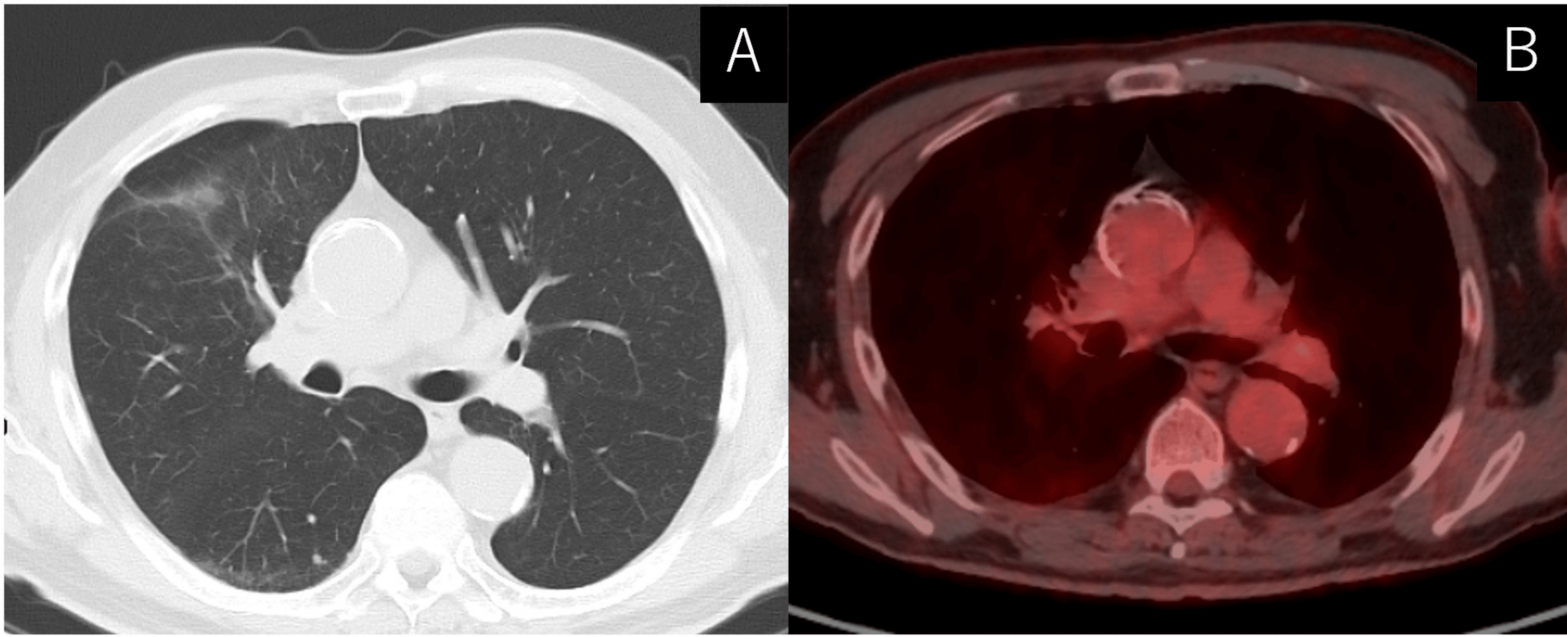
Radiological image after treatment (A) Computed tomography (CT) revealed shrinkage of mass and lymph node three years after treatment initiation. (B) Positron-emission tomography (PET)-CT revealed no uptake neither in shrunk mass or mediastinum.
